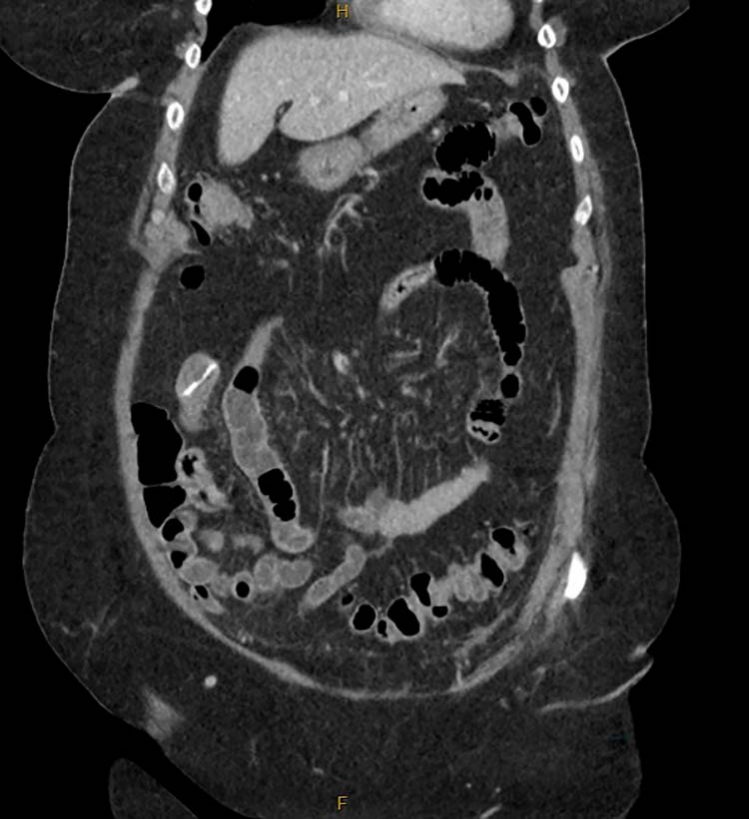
Initial CT imaging for patient RF.
